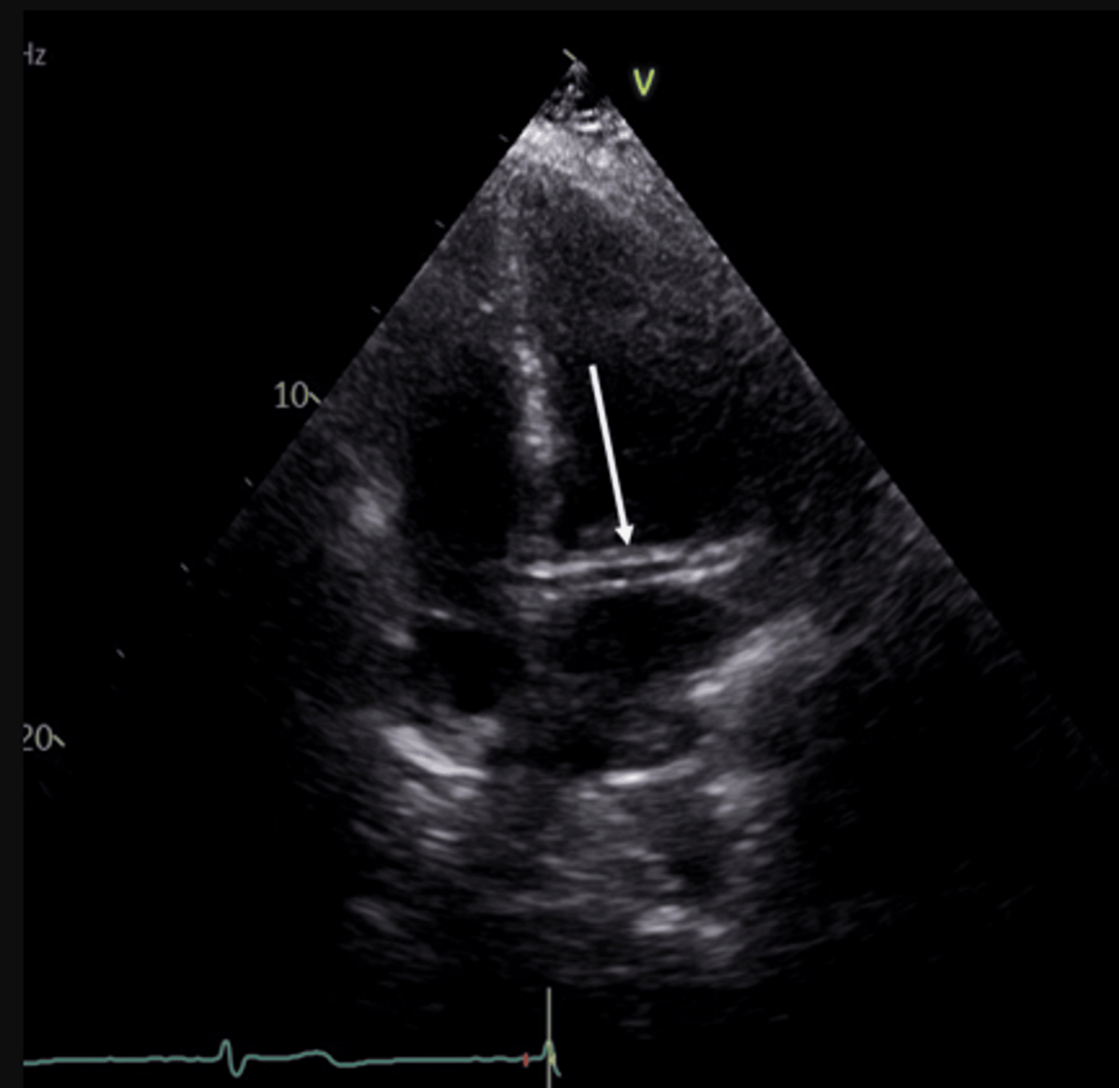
Transthoracic echocardiogram four-chamber view showing the tubular echogenic density parallel to the mitral valve (arrow).
